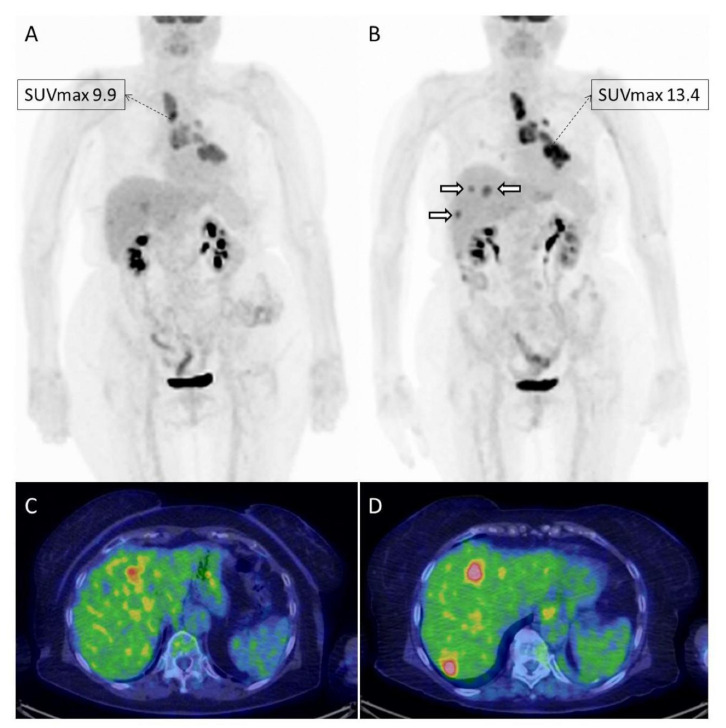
Example of a 78-year old female with advanced NSCLC treated with nivolumab and imaged with [^18^F]FDG PET/CT at baseline (A,C) and after 4 cycles of therapy (B,D). The patient resulted in overall stable on morphological imaging performed prior to PET/CT, which on the contrary documented a progressive metabolic disease. In fact, the tumor had an increase in metabolism (SUVmax and MTV), and showed the appearance of new lesions in the liver ((B); white hollowed arrows), only partially detectable on baseline imaging.
